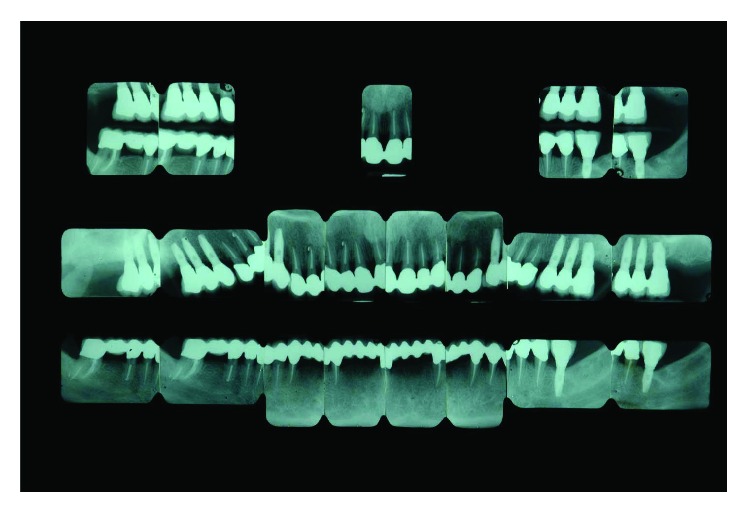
Final case: full-mouth intraoral radiographic exam (May 2002).
